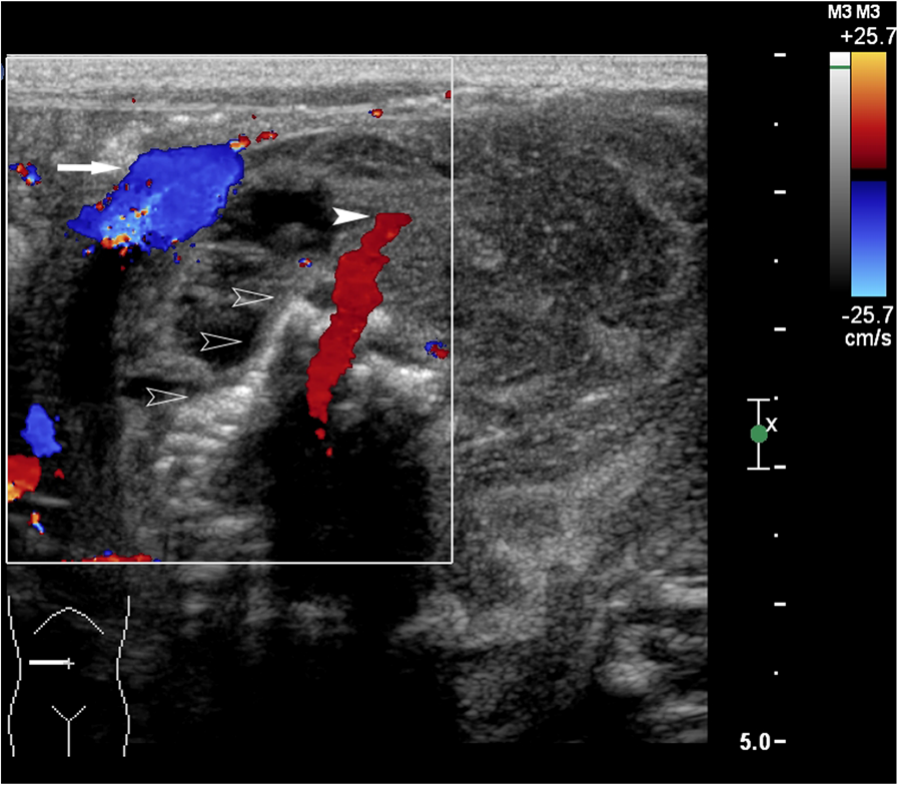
Transverse color Doppler ultrasound imaging: Ultrasound showing an abdominal aorta-like structure (white arrowhead) and an umbilical vessel-like structure (white arrow) within the abdominal mass. Multiple separated, small hyperechoic spots can also be seen, highly organized in a vertebral column-like structure (hollow arrowhead)
